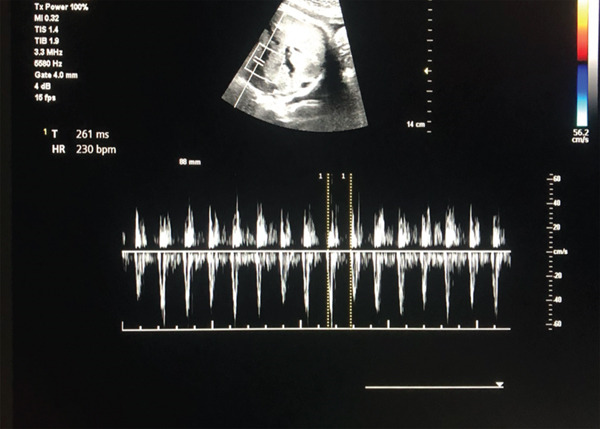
Pulse wave Doppler of aortic outflow on fetal echocardiography in a fetus at 35 weeks and 5 days of gestational age, demonstrating a heart rate of 230 bpm, at the time of admission to the hospital.
